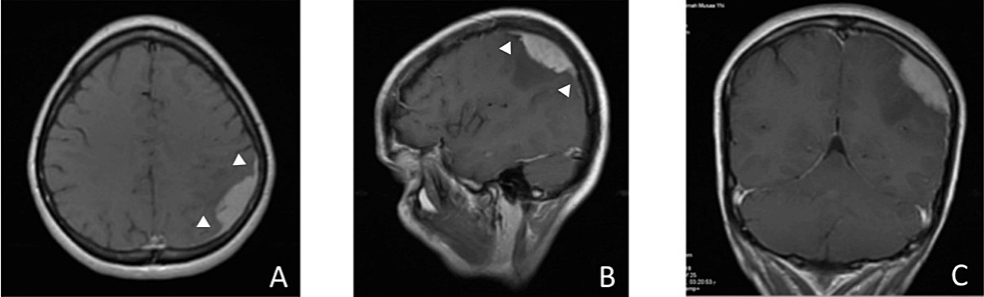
Preoperative magnetic resonance imaging (MRI) Axial (A), sagittal (B), and coronal (C) views of T1-weighted brain MRI show a homogeneously enhancing lesion surrounded by edema in the left parietal region. Note the dural tail sign (white arrowhead).
